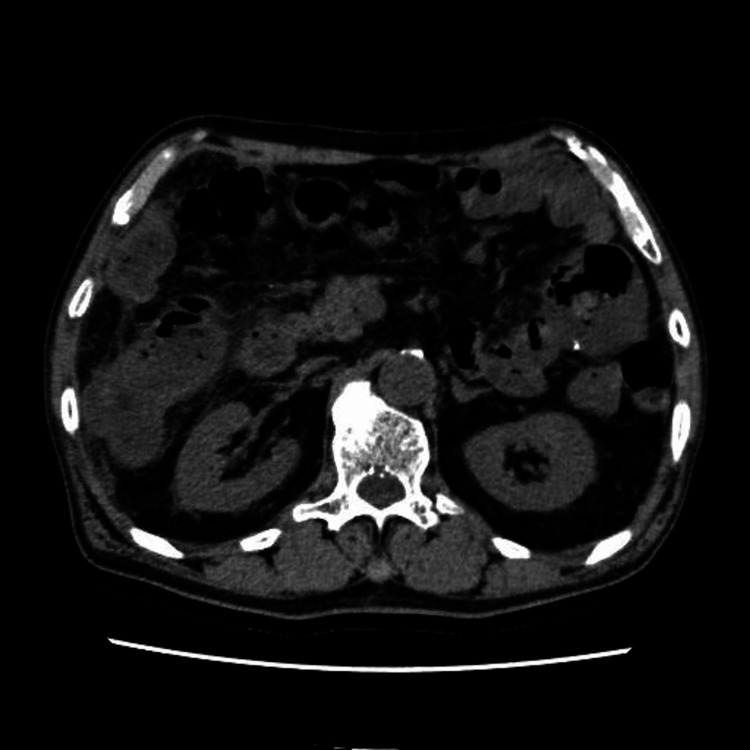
Computed tomography image of suspected bowel obstruction due to the thickening of the colon wall at the hepatic flexure.
